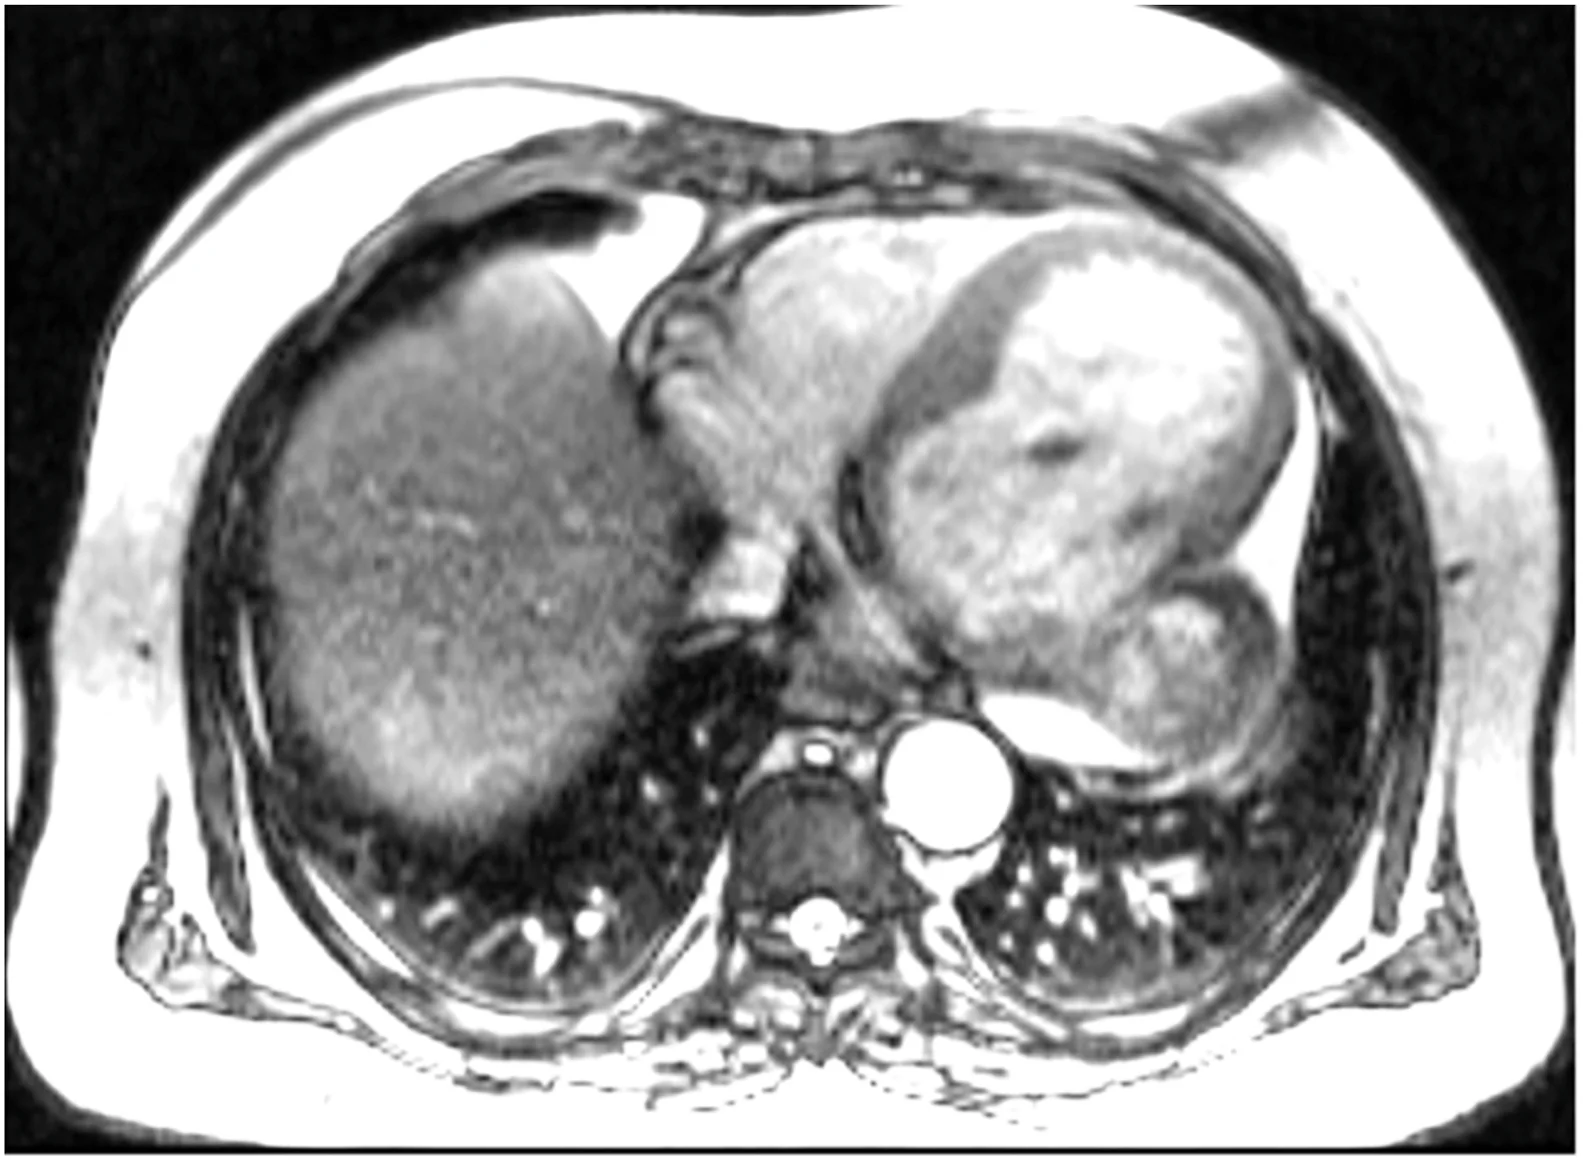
Preoperative cardiac magnetic resonance imaging showing a large left ventricular pseudoaneurysm arising from the anterolateral wall.
